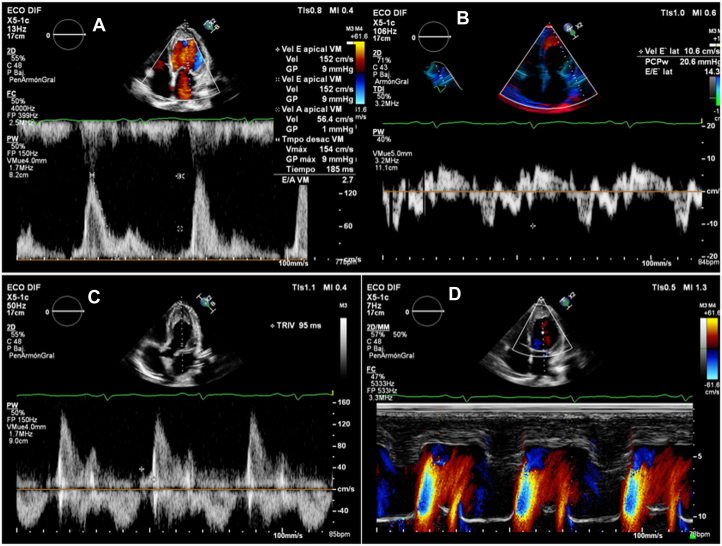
Echocardiographic Evidence of Restrictive Physiology in Eosinophilic Cardiomyopathy (A) Pulsed-wave Doppler at the mitral inflow showing a restrictive filling pattern with increased early diastolic velocity (E-wave 152 cm/s), reduced atrial contraction velocity (A-wave 56 cm/s), and a shortened deceleration time (185 ms), consistent with elevated left ventricular filling pressures (*E/A* = 2.7). (B) Tissue Doppler imaging (TDI) of the lateral mitral annulus demonstrating reduced early diastolic velocity (*E'* = 10.6 cm/s) and an elevated *E*/*E'* ratio (14.3), confirming increased filling pressures (PCPw 20.6 mm Hg). (C) Continuous-wave Doppler across the mitral valve showing a shortened isovolumic relaxation time (95 ms). (D) Color M-mode Doppler demonstrating rapid early diastolic transmitral flow propagation, characteristic of restrictive left ventricular physiology.
